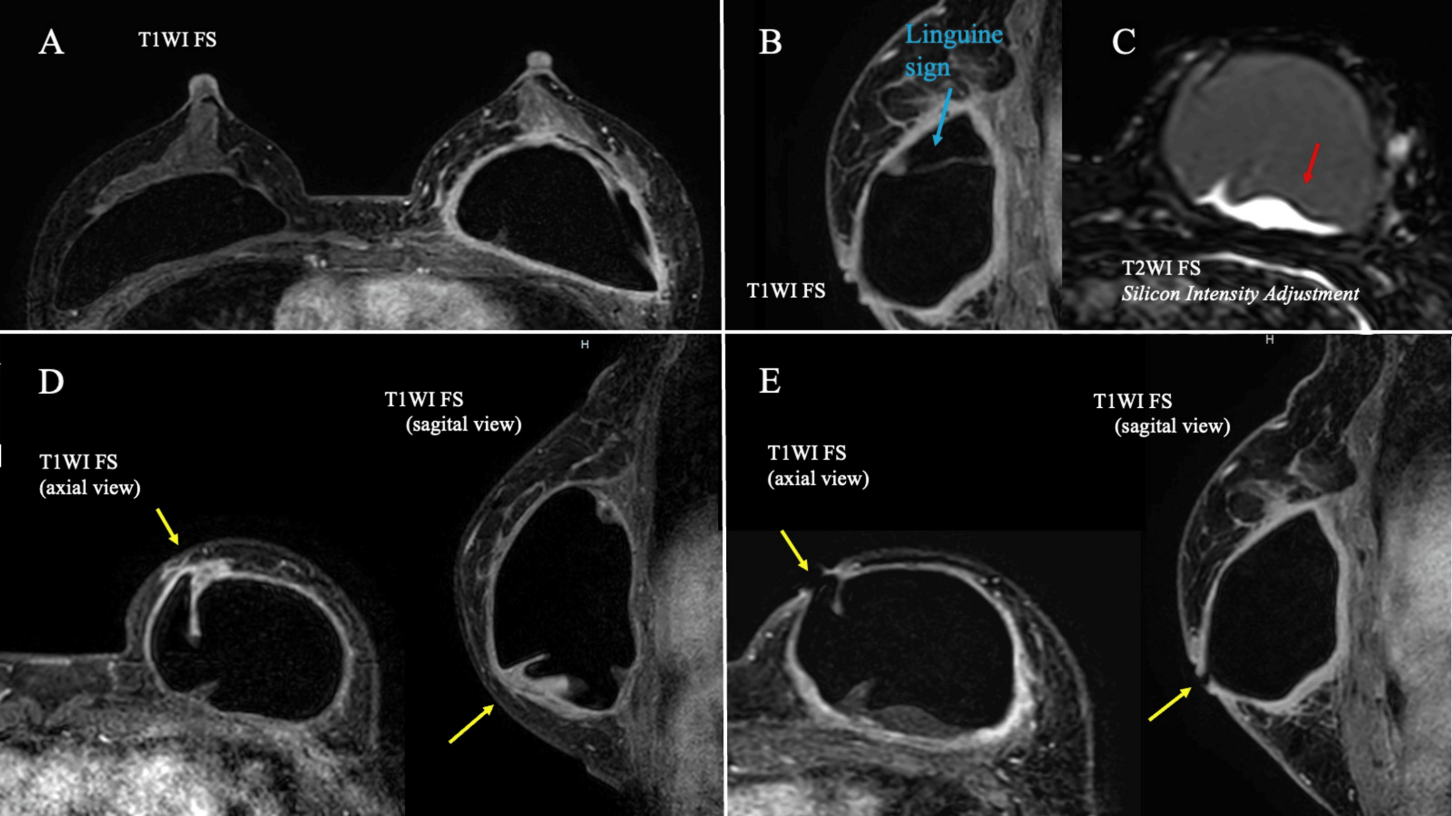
MRI findings. (A) A contrast-enhanced MRI showed enhancement around the SBI. (B) The sagittal view using T1WI with FS showed the linguine sign (blue arrow). (C) The silicone-enhanced T2WI image showed an area of high signal within the SBI (red arrow). (D) The site of the skin ulcer was observed three years ago, showing deformation of the SBI (yellow arrow). (E) Silicone was exposed at the site of the skin ulcer (yellow arrow) FS: fat suppression; MRI: magnetic resonance imaging; SBI: silicone breast implant; WI: weighted image
